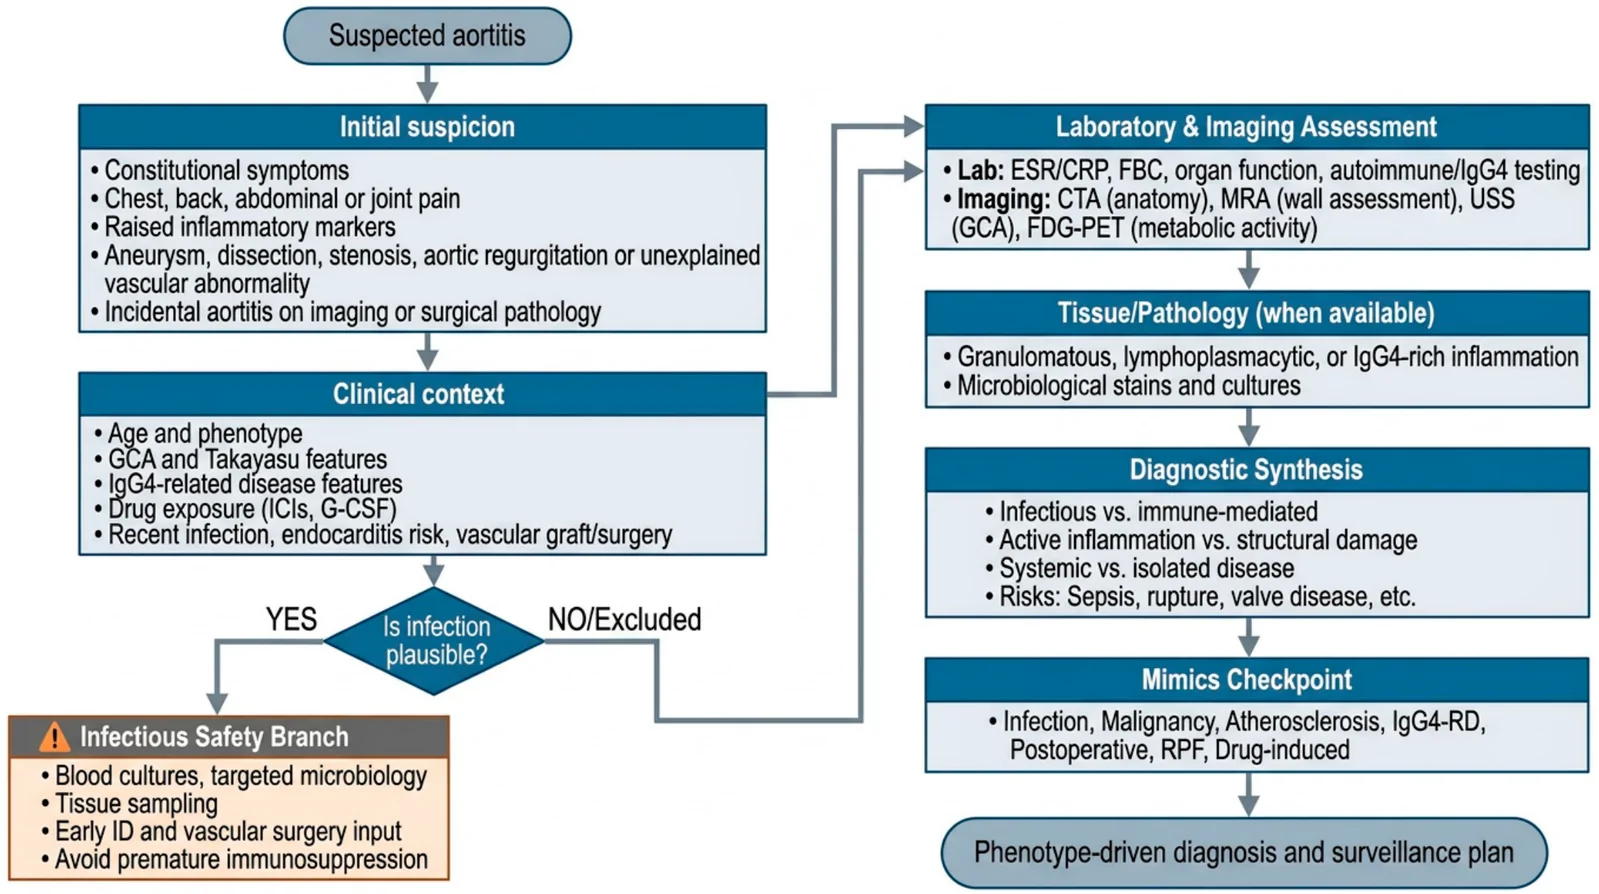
Diagnostic algorithm for suspected aortitis. The pathway emphasises clinical suspicion, infection exclusion, laboratory assessment, multimodality imaging, tissue assessment where available, and reassessment when clinical, microbiological, serological or imaging findings are discordant. Abbreviations: CRP, C-reactive protein; CTA, computed tomography angiography; ESR, erythrocyte sedimentation rate; FBC, full blood count; FDG-PET, fluorodeoxyglucose positron emission tomography; GCA, giant cell arteritis; G-CSF, granulocyte-colony stimulating factor; ID, infectious diseases; ICI, immune-checkpoint inhibitor; IgG4, immunoglobulin G4; IgG4-RD, IgG4-related disease; MRA, magnetic resonance angiography; RPF, retroperitoneal fibrosis; USS, ultrasound scan.
